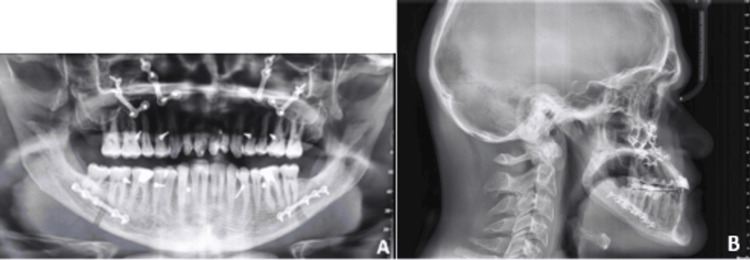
Postoperative radiographic records (A) Postoperative panoramic radiograph. (B) Post-surgical lateral cephalometric radiograph
